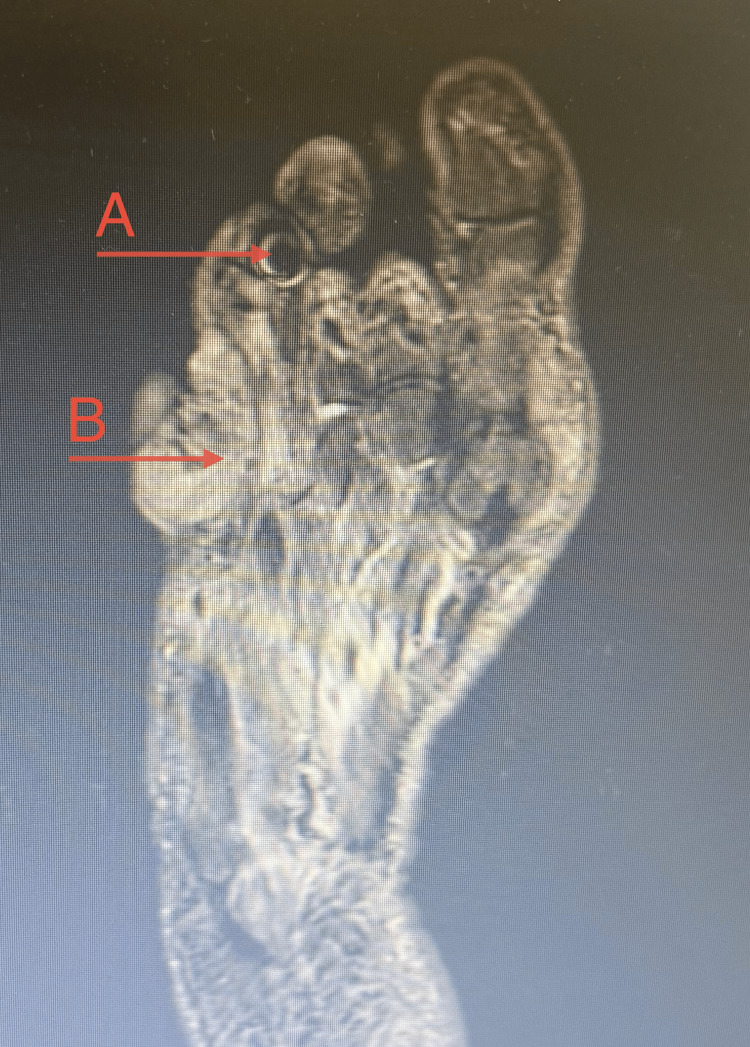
Coronal short tau inversion recovery (STIR) MRI of the lower limb demonstrating a metallic foreign body associated with surrounding phlegmon A: Metallic inclusion B: 37 × 17 mm, enhancing soft tissue lesion in the interdigital space, involving the subcutaneous tissues
